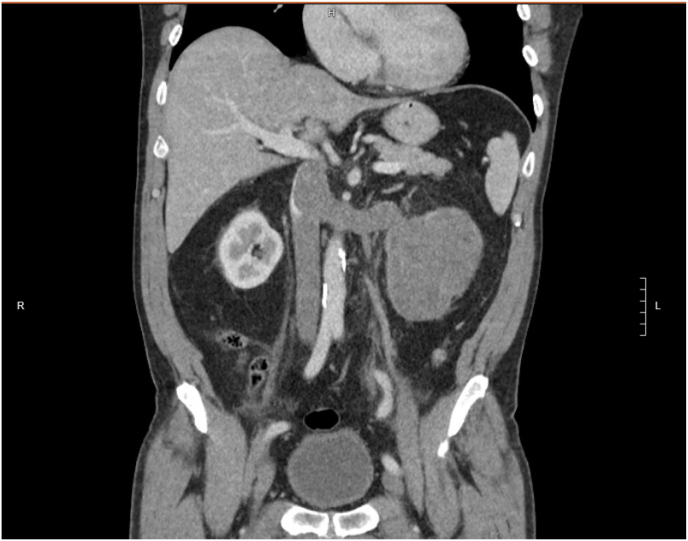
CT scan of abdomen and pelvis showing left renal mass with tumor thrombus in the renal vein and IVC.
